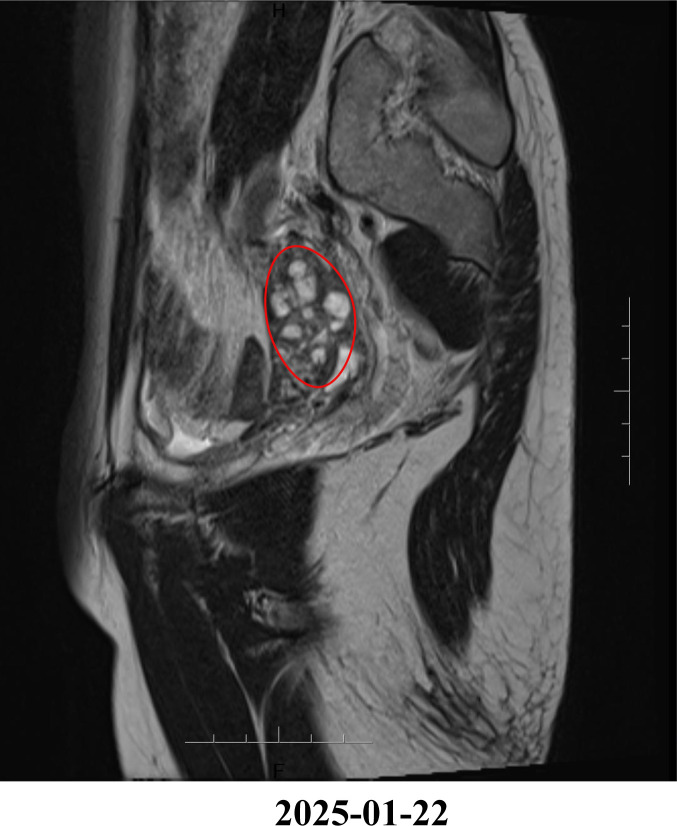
Pelvic MRI in January 2025. Sagittal T2-weighted fast spin-echo sequence (TE 83 ms, TR 5990 ms, slice thickness 4.0 mm) showing multiple small cystic lesions in the left adnexal region.
